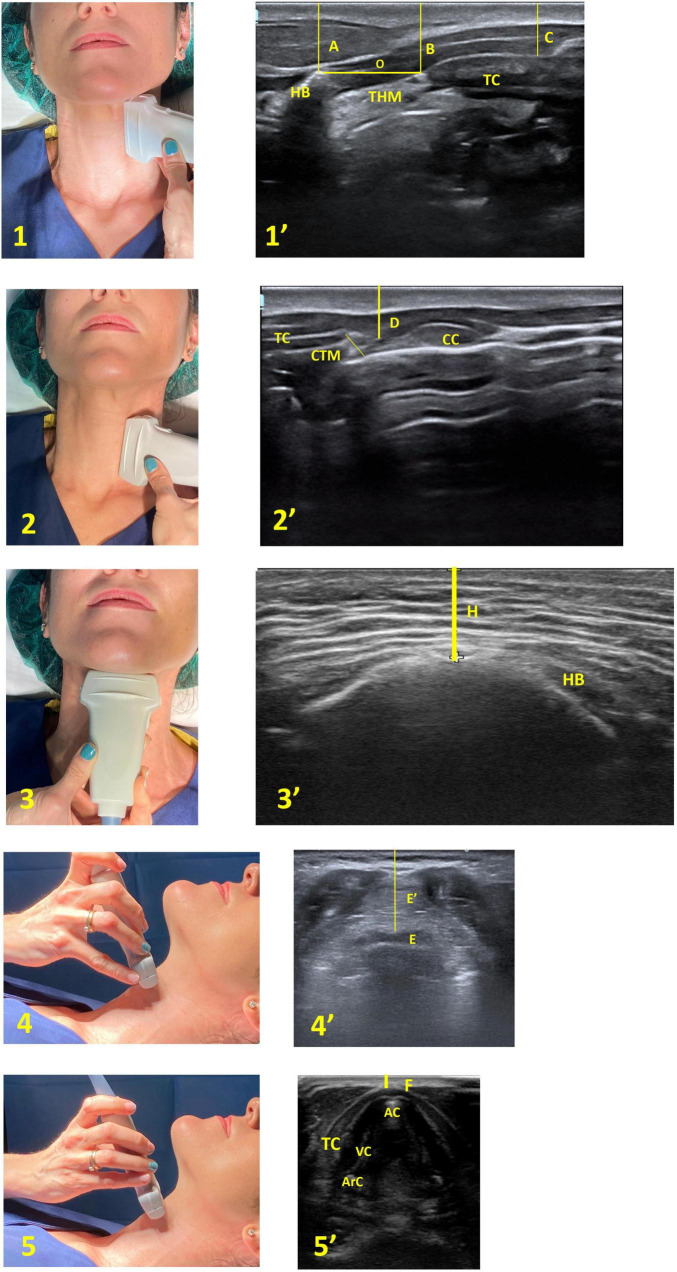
Ultrasound measurements of pre-tracheal tissues. Left panel different neck levels with ultrasound probe. (1) and (2) patient in sniffing position and probe in parasagittal plane. (3) (4) and (5) probe in transverse plane. (1): Thyrohyoid membrane level; (2): cricothyroid membrane level; (3): Hyoid bone level; (4): Epiglottis level; (5): Thyroid cartilage level. Right panel corresponding ultrasound images: (1’): (HB) Hyoid bone (THM) Thyrohyoid membrane (TC) Thyroid cartilage (A) Measure the distance from the hyoid bone to the skin (B) Distance from the thyrohyoid membrane to the skin (C) Distance from the thyroid cartilage to the skin (O) Distance from hyoid bone to thyroid cartilage. (2’): (TC) Thyroid cartilage (CTM) Cricothyroid membrane (CC) Cricoid cartilage (D) Measure the distance from the cricothyroid membrane to the skin. (3’): (HB) Hyoid bone (H’) Distance from Skin to Hyoid Bone. (4’): (E) Epiglottis (E’) Measure the distance from the epiglottis to the skin. (5’): (AC) Anterior commissure (TC) Thyroid cartilage (VC) Vocal cords (ArC) Arytenoid cartilage (F) Distance from skin to thyroid cartilage.
